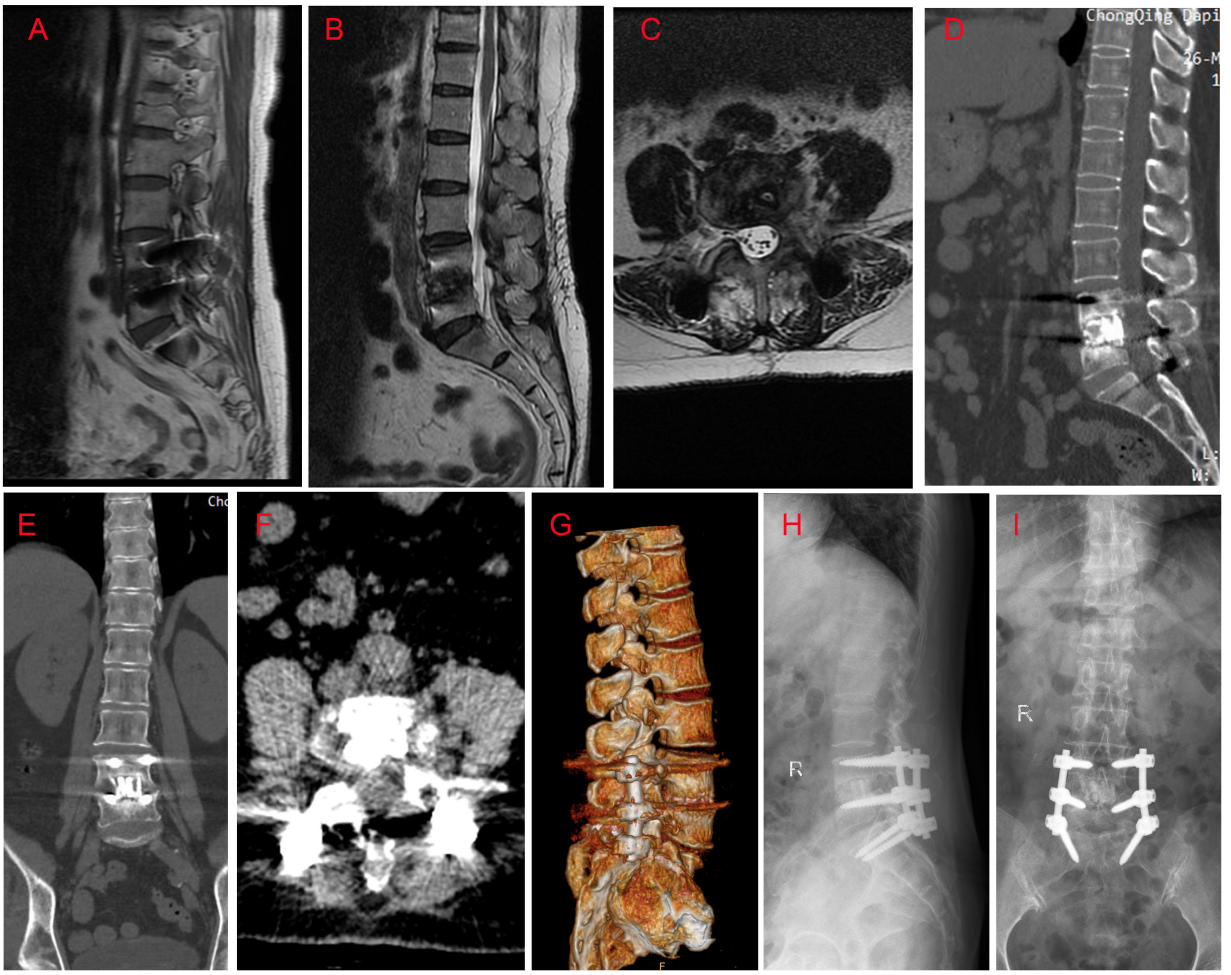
MRI, CT and X ray findings at six month follow-up. (A) Sagittal T1-weighted image; (B) Sagittal T2-weighted image; (C) Axial T2-weighted image at the level of L4/5; (D) Sagittal CT image; (E) Coronal CT image; (F) Axial CT image; (G) Lateral X ray image; (H) Anteroposterior X ray image; (I) CT three-dimensional reconstruction image. Imaging findings revealed that: the pedicle screws and cages were inserted in the right place, without cracked or displacement; there was no evidence of spinal infection; a certain level of L4/5 interbody fusion was observed.
